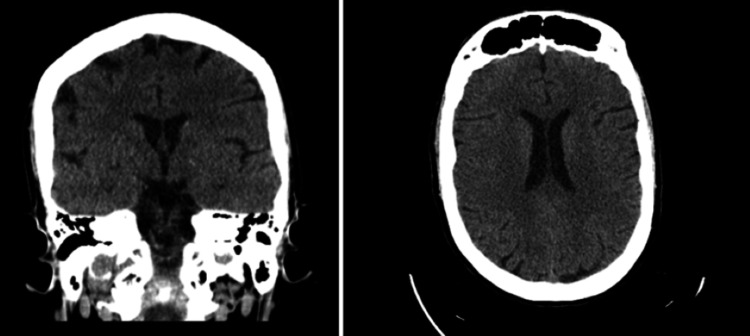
CT head without contrast showed no significant abnormality.
